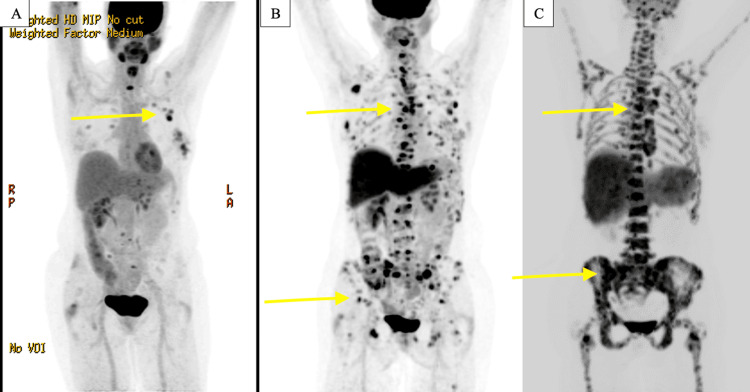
PET/CT scans performed at different points during the evolution of the disease. (A) In December 2020, there was increased fluorodeoxyglucose (FDG) activity in the left breast, axillary lymph nodes, and spine. (B) In June 2021, there was a worsening of metabolic activity in the thoracolumbar spine and pelvic bones. (C) In February 2022, there was further progression given the presence of multiple mediastinal and abdominal lymphadenopathy as well as extensive metastasis in the axial and appendicular skeleton.
